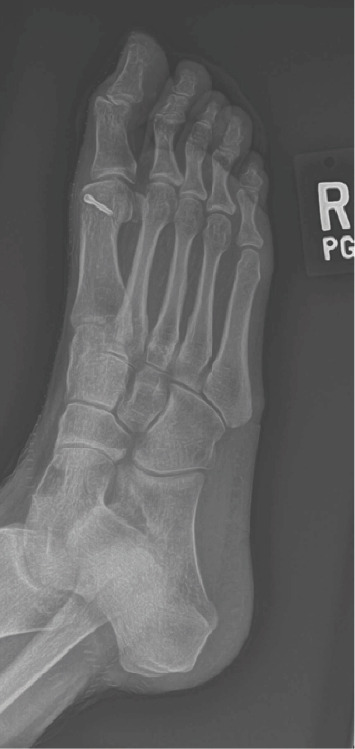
Postoperative oblique radiograph of the right foot demonstrating hallux rigidus correction with OCA implantation on the metatarsal head.
